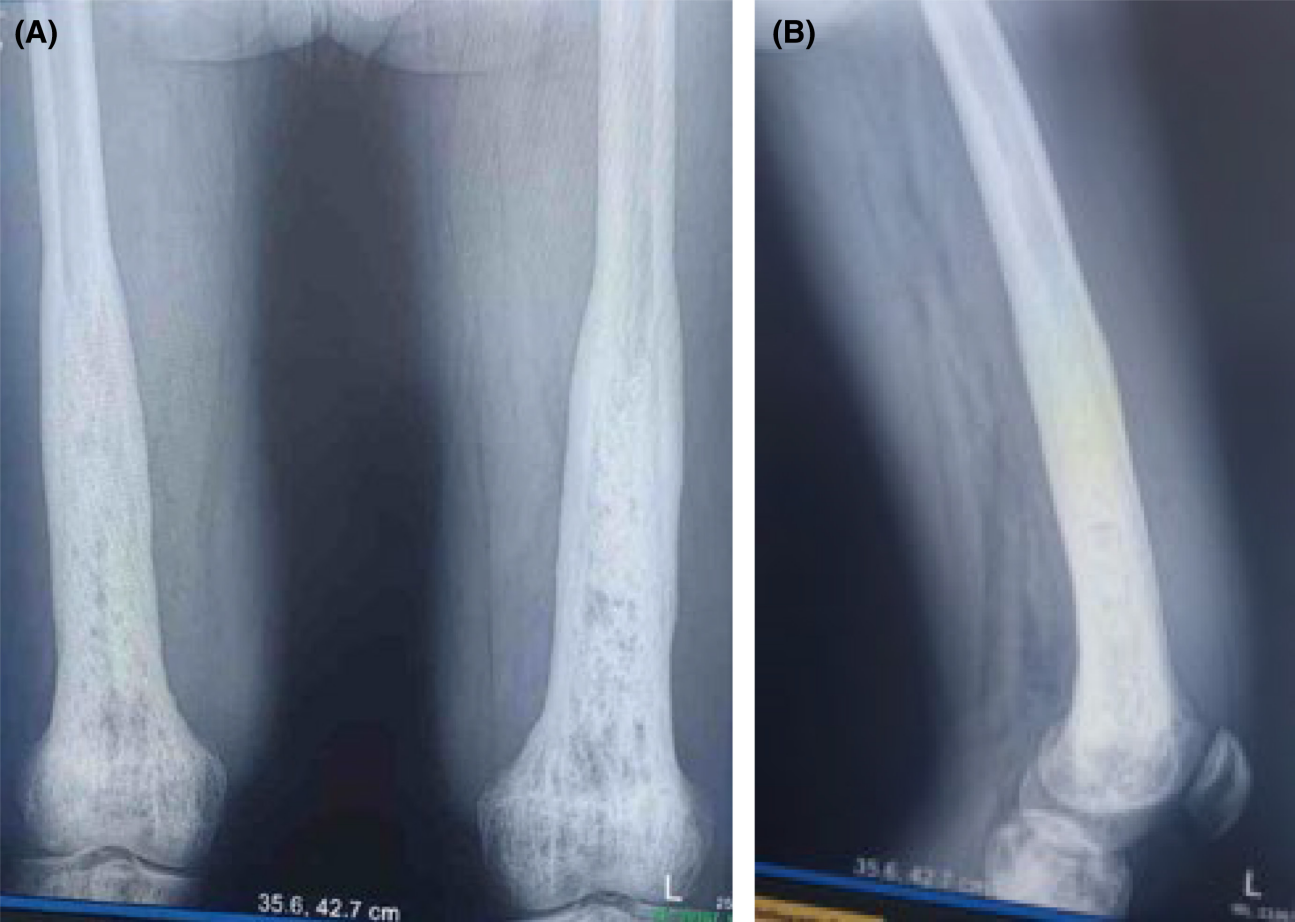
Frontal (A) and lateral (B) radiography of the femur shows bilateral symmetric distal metadiaphyseal cortical thickening and diffuse medullary sclerosis with loss of corticomedullary differentiation.
